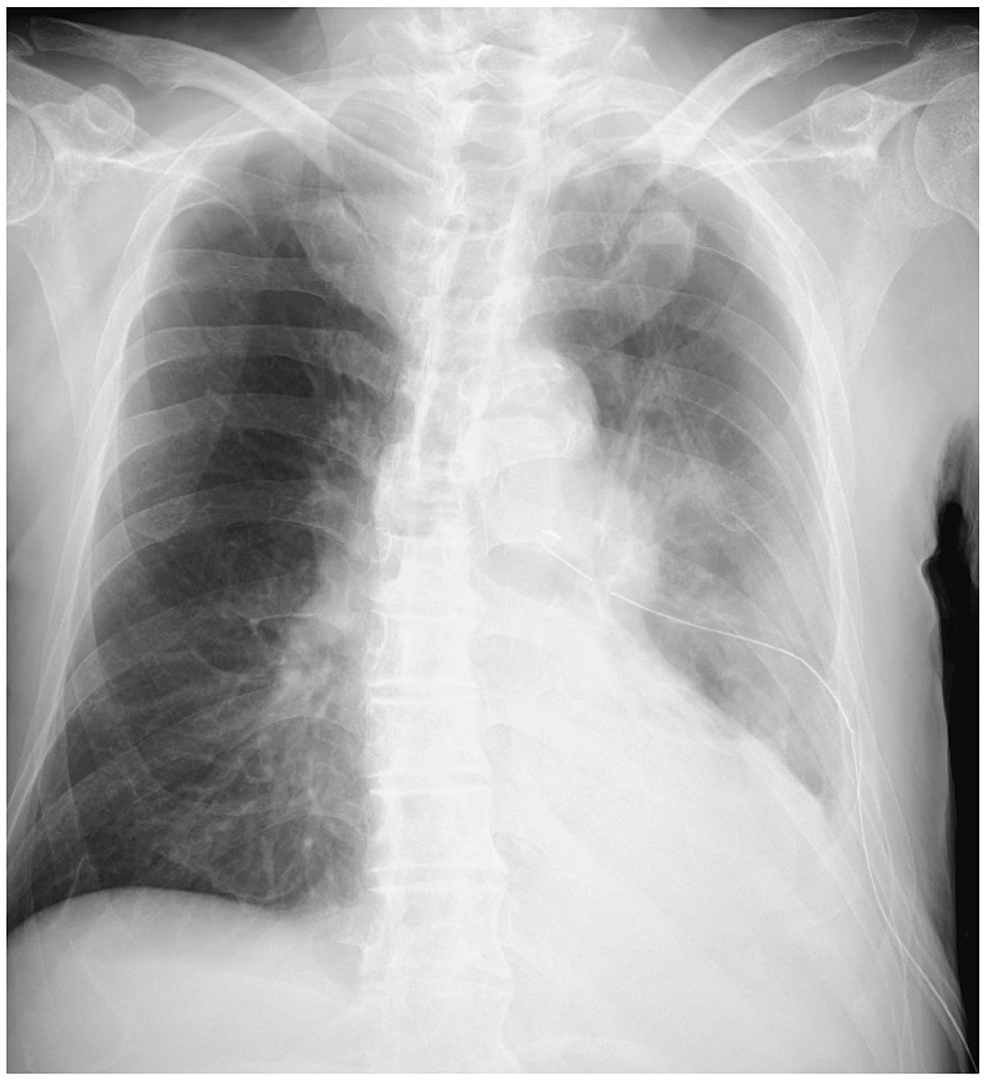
Preoperative chest radiography finding The chest tube was inserted through the left seventh intercostal space. Hazy opacities and pleural effusion were observed in the left middle and lower lung zones.
